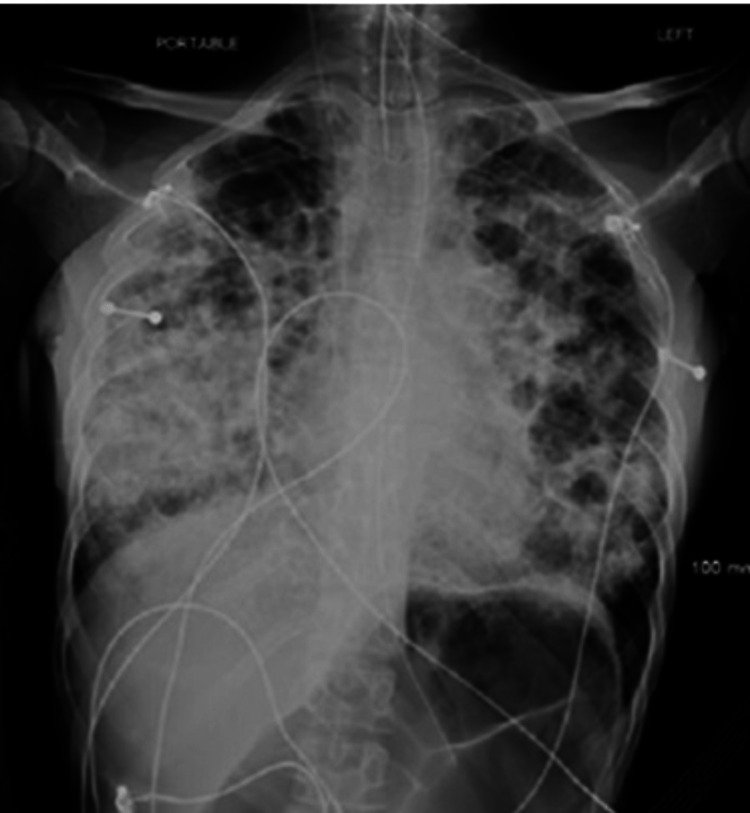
ICU chest X-ray with extensive cavitation and consolidation A chest X-ray was taken in the ICU redemonstrating cystic bronchiectasis and severe cavitary disease with extensive consolidations bilaterally.
